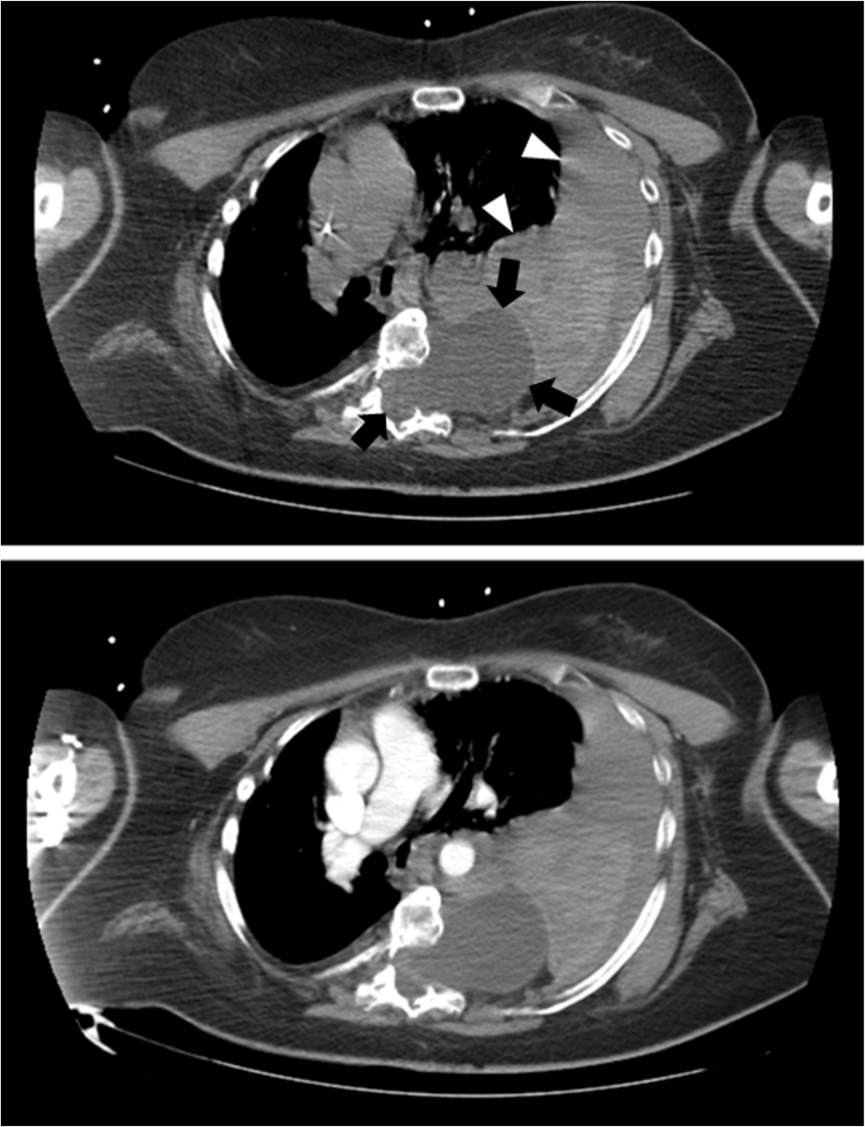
Chest computer tomography (CT). Upper figure: without contrast enhancement. Lower figure: with contrast enhancement. The arrows show the intrathoracic meningocele and the arrowheads show the hemothorax.
